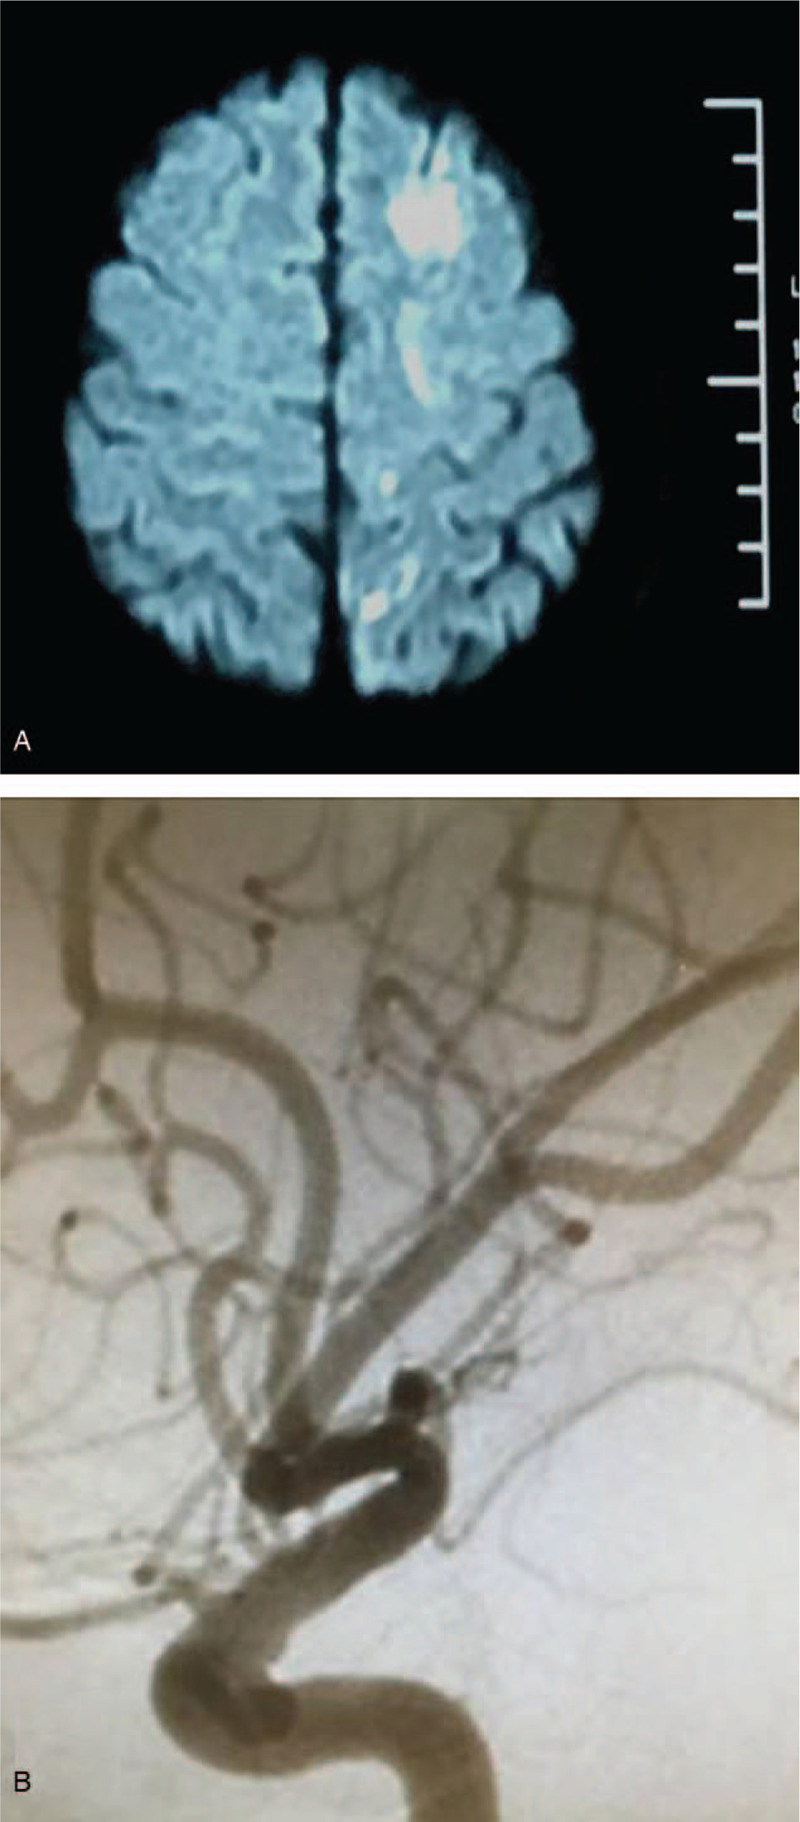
Diffusion-weighted magnetic resonance imaging (MRI-DWI), digital subtraction angiography (DSA). (A) MRI-DWI showed acute ischemic stroke in the left frontal and parietal lobe. (B) DSA showed unruptured intracranial aneurysm on supraclinoidal segments of left internal carotid artery.
